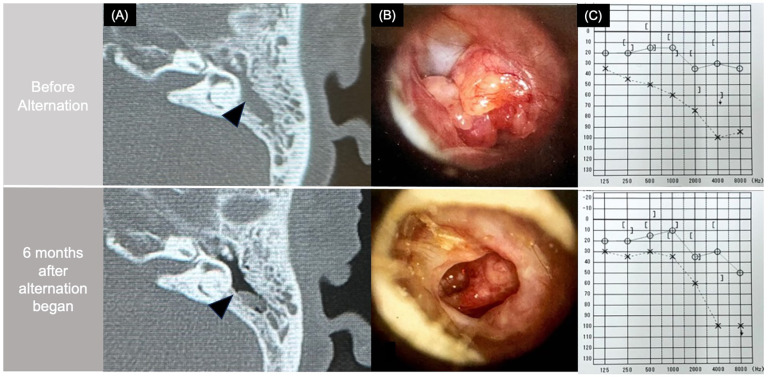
Computed tomography (CT), otoscopic, and audiometric changes before and after alternating therapy. (A) CT scan showing middle ear opacification before therapy (top) and resolution after therapy (bottom). (B) Otoscopy demonstrating reduction of granulation tissue after treatment. (C) Audiometry showing hearing improvement following alternating therapy.
